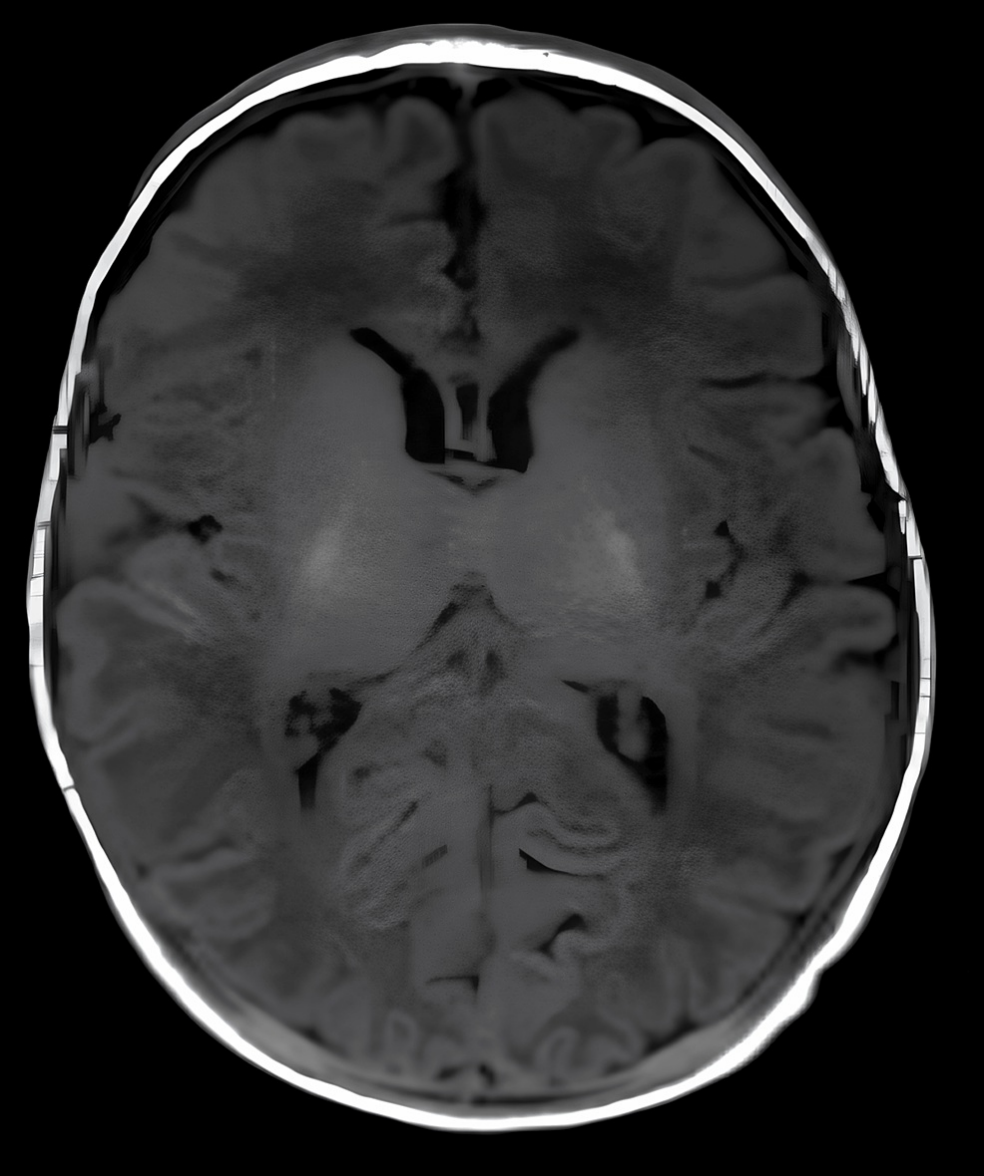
MRI brain at three weeks; T1-weighted image showed a normal structure of the brain. MRI, magnetic resonance imaging
